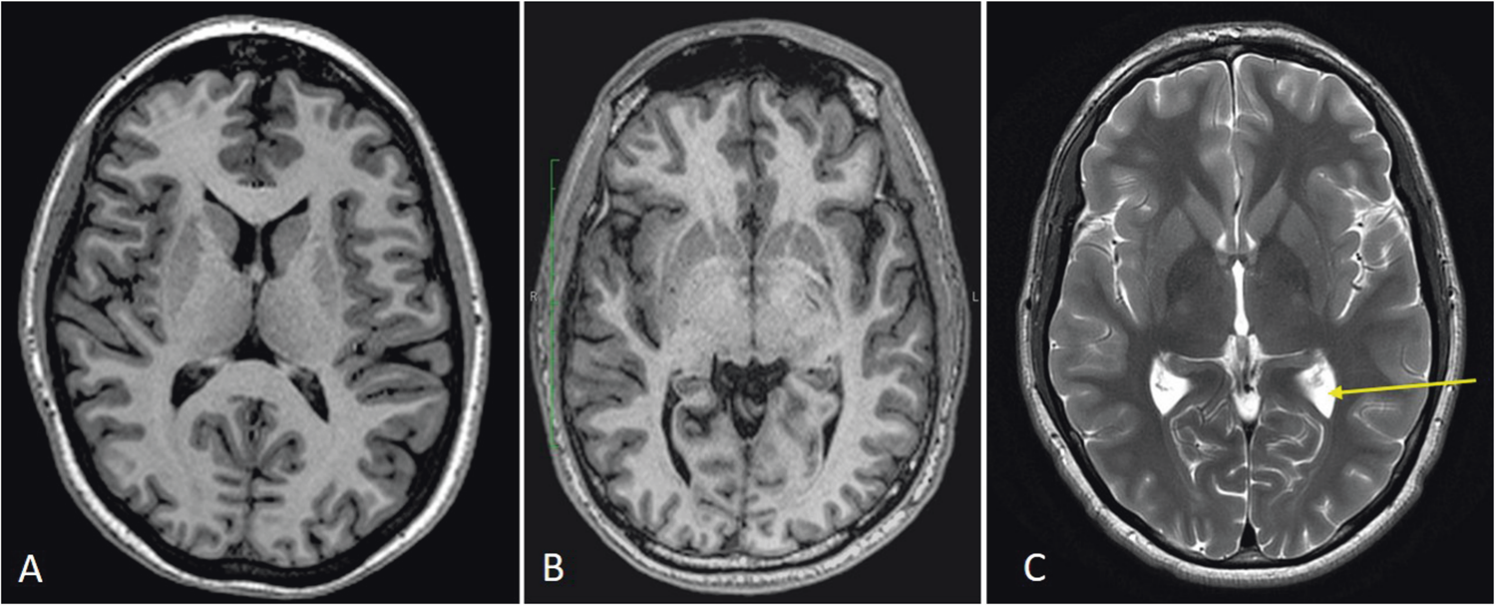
Structural MRI. T1 (A, B) and T2 (C) weighted images are two main types of image contrast used to characterize tissue and structures in MRI. A and B show images from a T1w MPRAGE sequence. A is a traditional T1w MPRAGE that clearly delineates the white and gray matter structures in the brain as shown in this axial image. White matter is brighter than gray matter on T1 weighted images. B is produced from the newer MPRAGE PROMO (PROspective MOtion correction) sequence, which provides the utility of reducing motion artefacts which can be problematic in some patients. C is produced from the T2w fast spin echo sequence that complements the T1w images. Fluid is bright on T2w images as demonstrated by the bright CSF in the ventricles (arrow). Gray and white matter are reversed with white matter being darker on T2w images.
